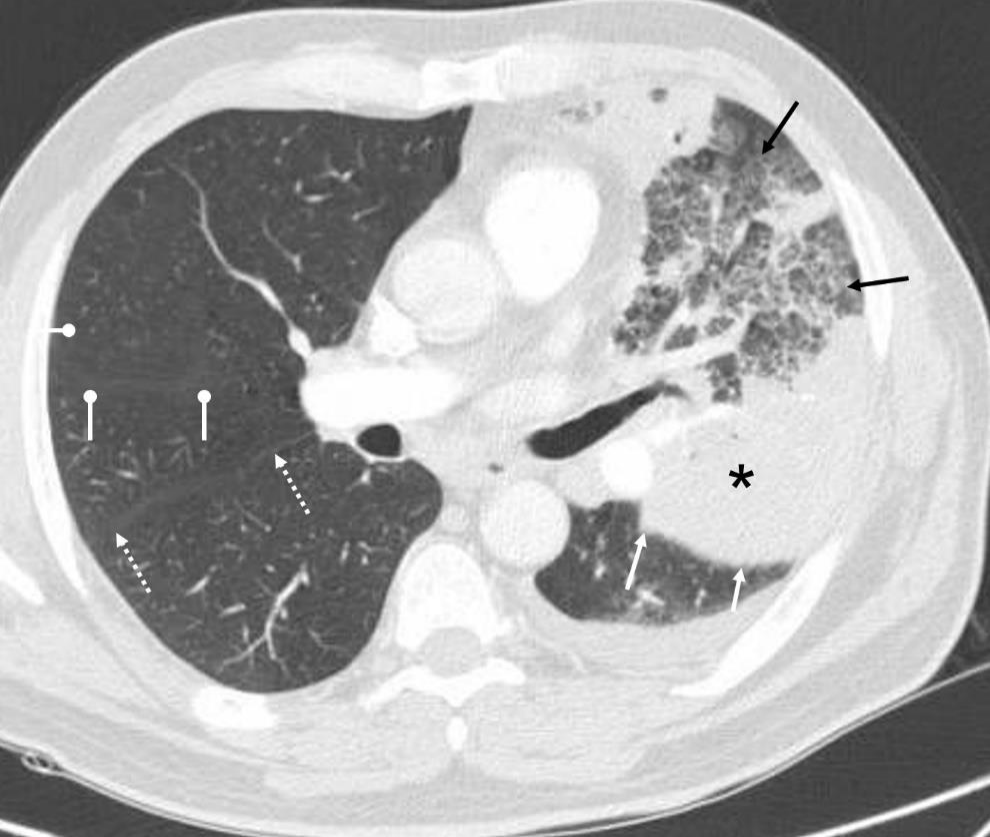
An axial CT image (lung window) of a 71 years old female with pneumonia shows a large area of homogeneously increased density suggesting consolidation (asterisk) and ill-defined heterogeneous areas of increased density suggesting glass opacities (solid black arrows) in the left upper lobe
